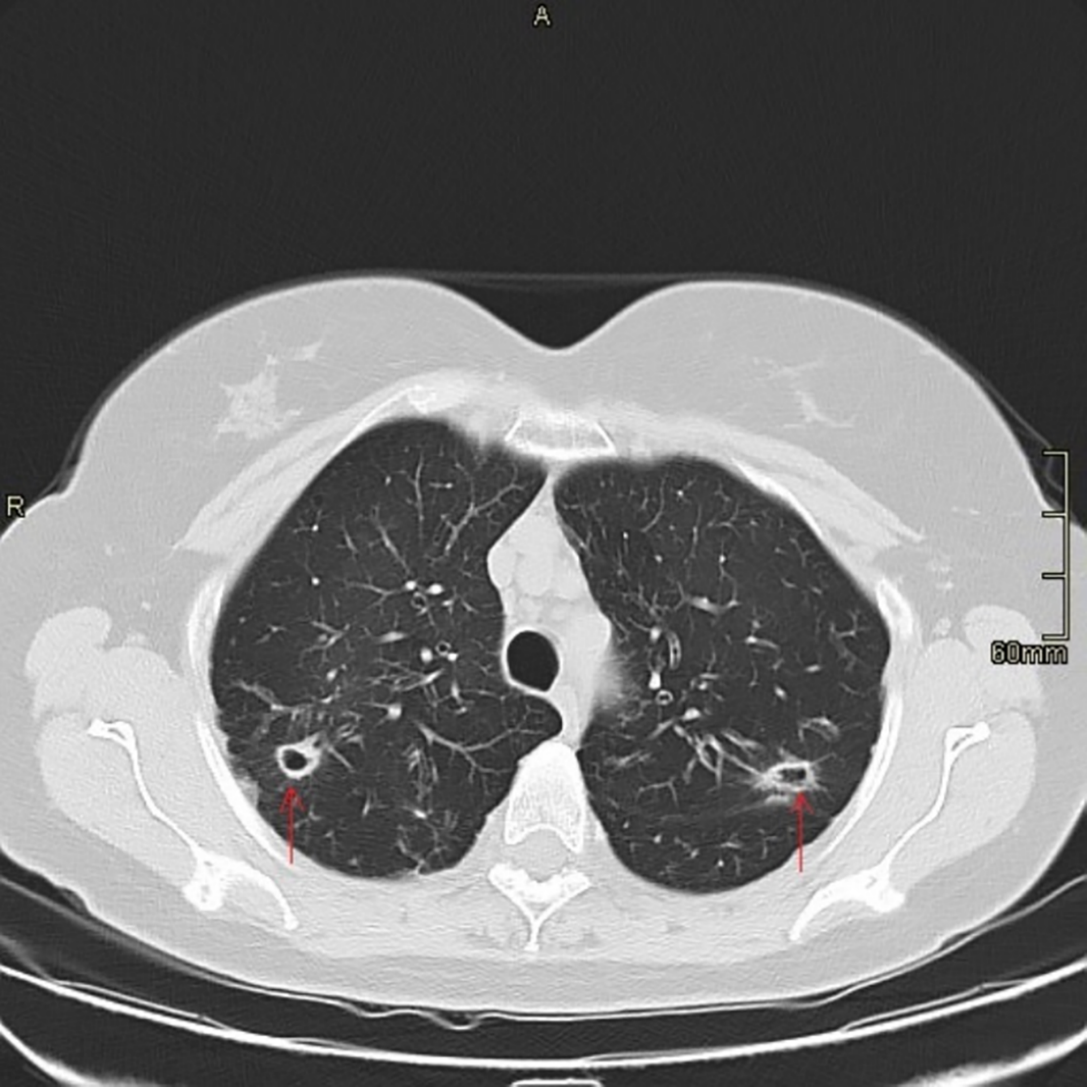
CT chest showing cavitary nodules.
